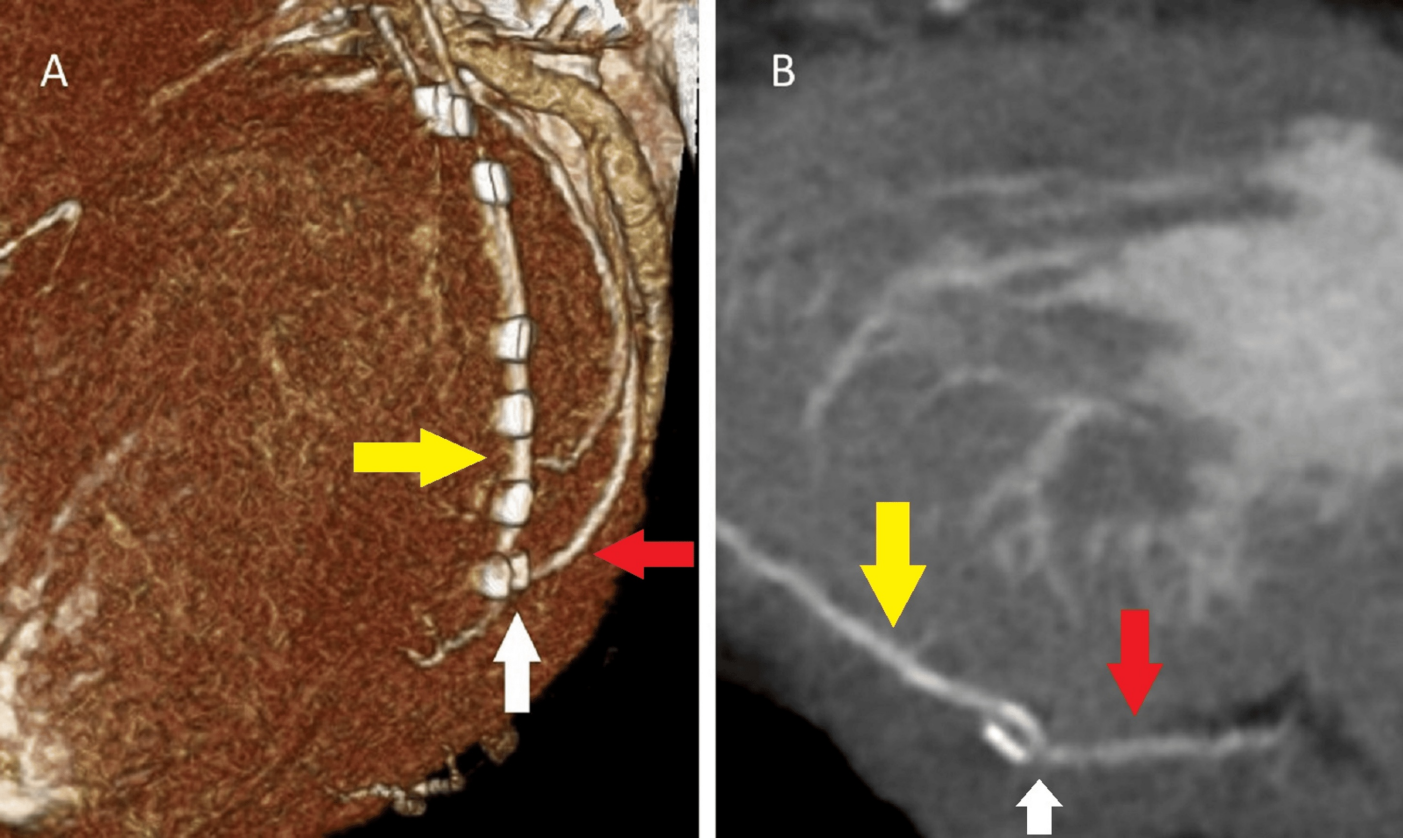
Contrast computed tomography (CT) performed on postoperative day 48 No pseudoaneurysm (white arrow) was observed at the anastomosis site of the left internal mammary artery (yellow arrow) to the circumflex artery (red arrow). A: Three-dimensional CT; B: Curved multi-planar reformation
